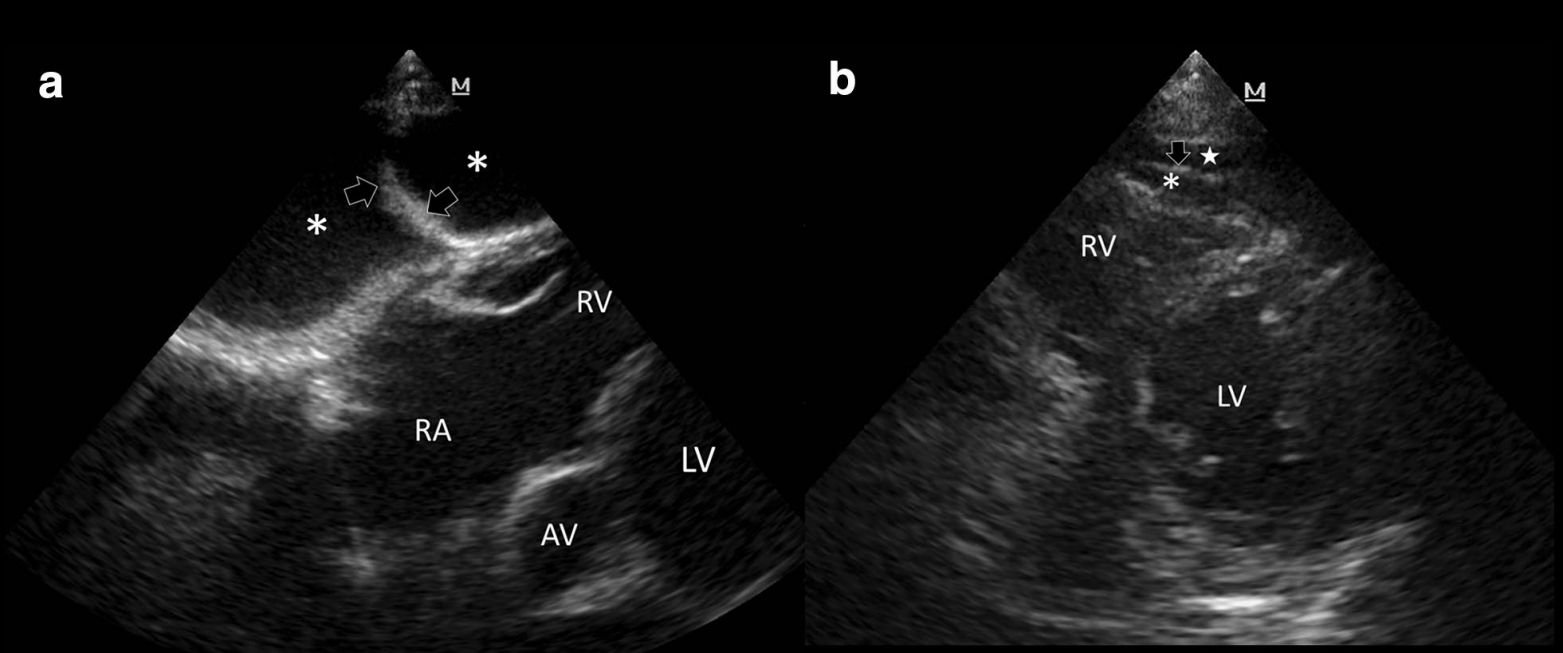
a Pericardial effusion versus ascites. Ascites (*asterisks*) is shown anterior to the heart in subcostal 4-chamber view. The falciform ligament (*arrows*) is depicted, confirming this effusion. *LV* left ventricle, *AV* aortic valve, *RV* right ventricle, *RA* right atrium. b Epicardial (*asterisk*) and mediastinal fat (*white star*), demonstrated in parasternal short-axis view, in systole; *LV* left ventricle, *RV* right ventricle, *arrow* pericardium
